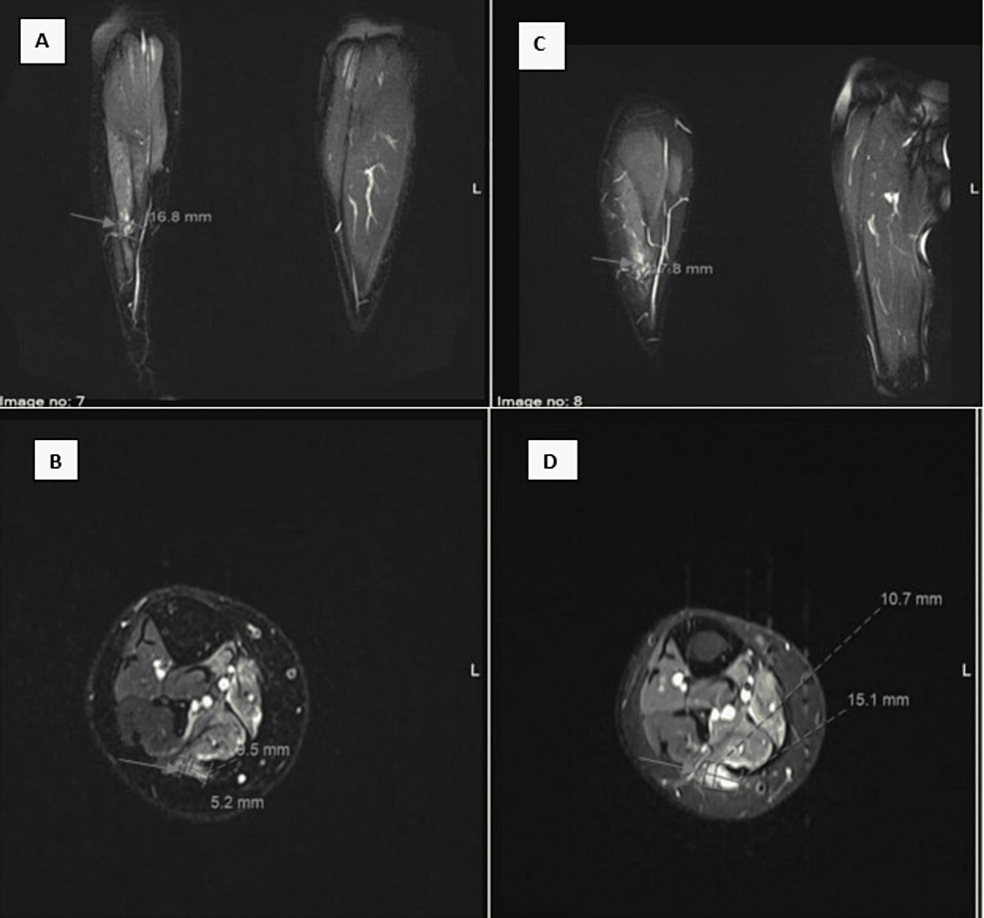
MRI right lower limb pre/post pazopanib A&B MRI right lower limb post pazopanib C&D MRI right lower limb pre pazopanib
